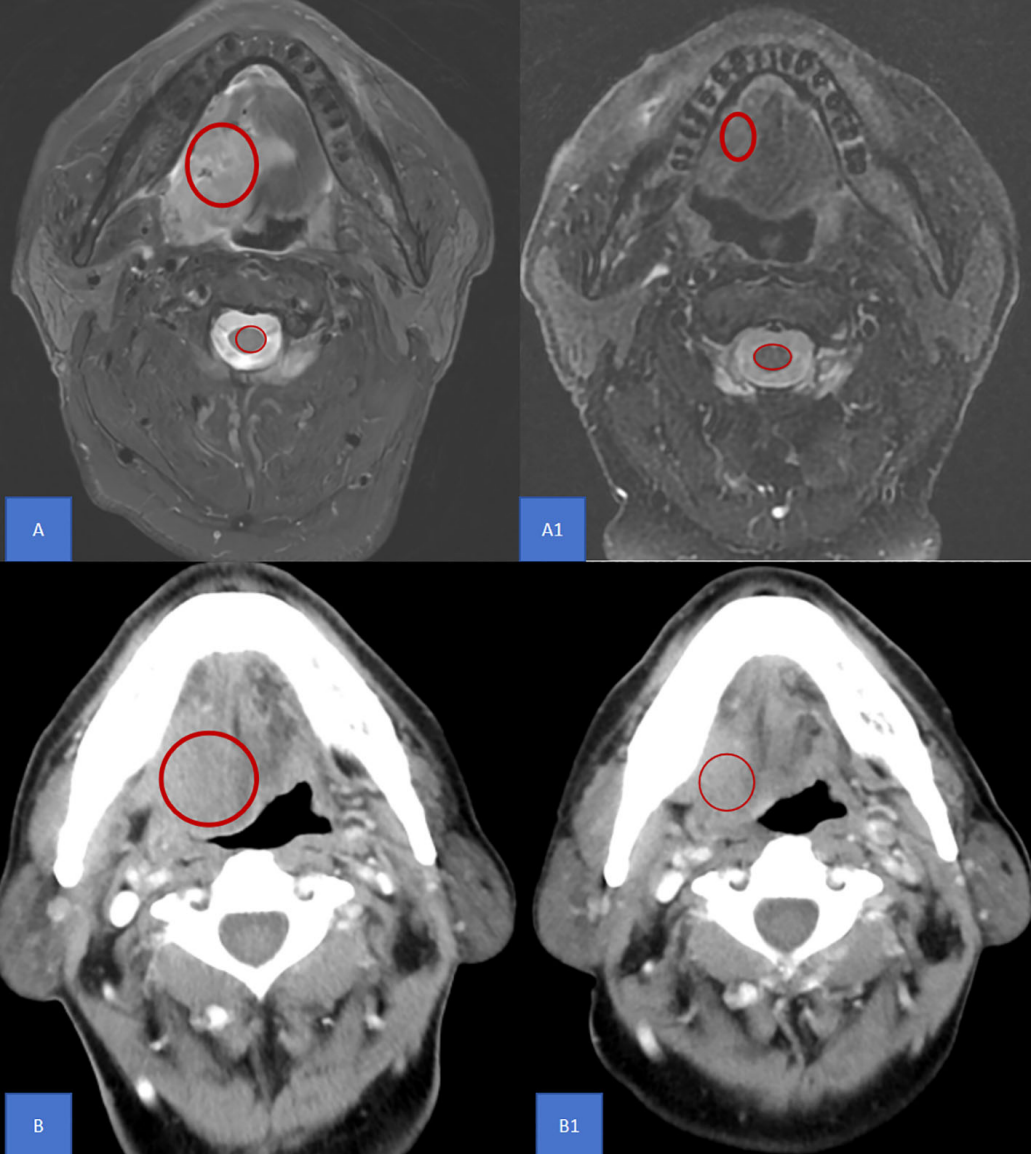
ROI definition The ROI average value was extracted from the maximum cross-sectional area of the tumor using a picture archiving and communication system as shown in the red circle. (A) A1 For enhanced MR, the ROI average value ratio of the T2-weighted image was calculated as the ratio of the relative ROI average value before NIC to that after NIC. The relative ROI average value was defined as the ratio of the ROI average value of the tumor to that of the spinal cord. (B) B1 For enhanced CT, the ROI average value ratio was calculated as the ratio of the ROI average value before NIC to that after NIC. MR, Magnetic resonance; CT, Computed tomography; AUC, Area under curve; ROI, Region of Interest; NIC, Neoadjuvant Immunochemotherapy.
